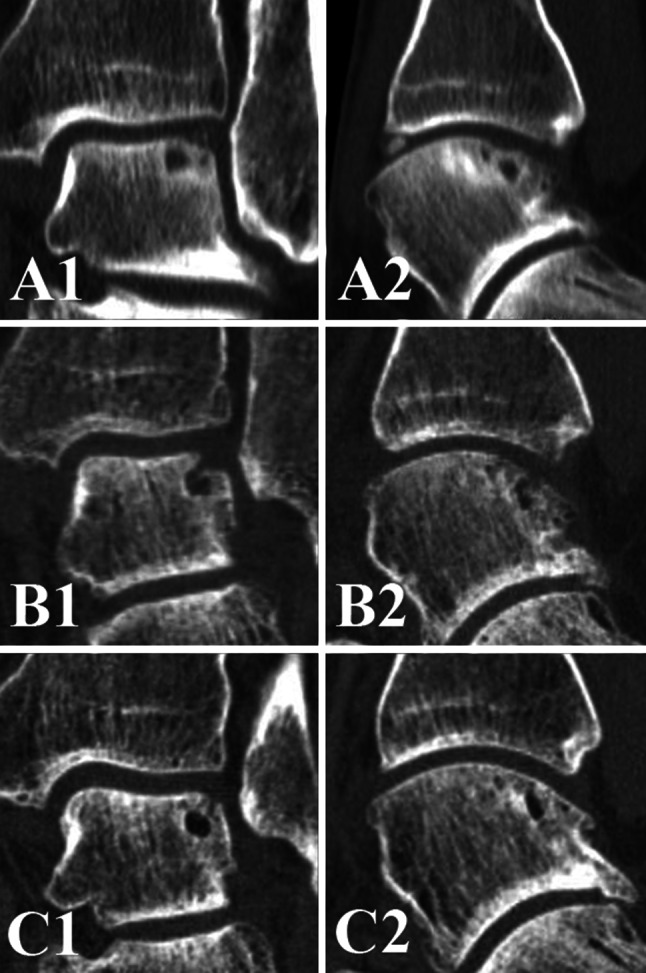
a Preoperative coronal (A1) and sagittal (A2) CT scans of a *left* ankle with a cystic OCD of the *lateral* talar dome, b the two weeks postoperative coronal (B1) and sagittal (B2) CT scans show that the cystic OCD is opened but not debrided, c at 1-year follow-up, the cyst is still visible [coronal CT scan (C1); sagittal CT scan (C2)]
